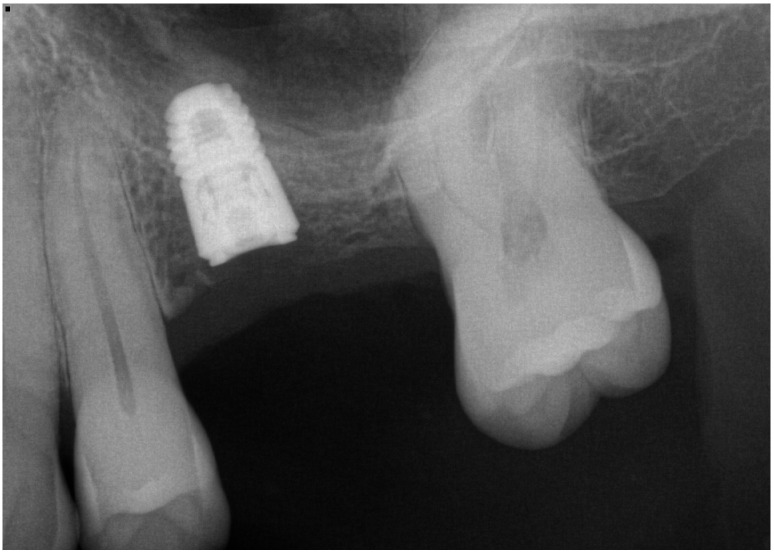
Periapical radiograph of Case #1 taken at the time of implant placement, demonstrating the elevation of the maxillary sinus.
